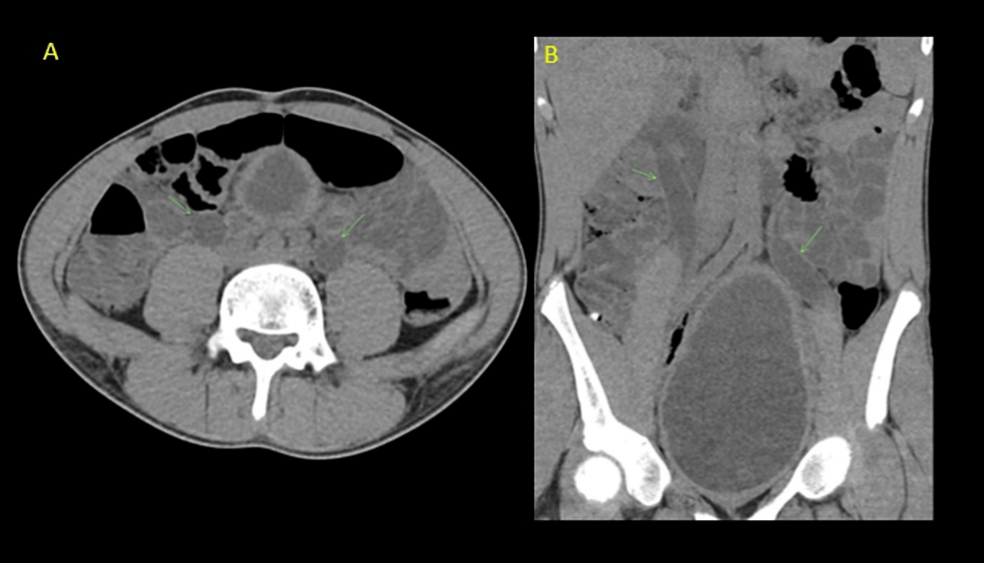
Computed tomography (CT) of the abdomen and pelvis Axial (A) and coronal (B) non-contrast CT images of the abdomen demonstrated bilateral hydroureteronephrosis (arrows).
